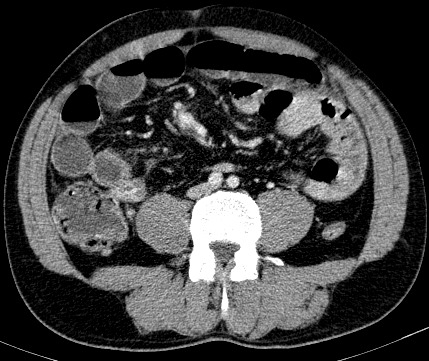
axial enhanced abdominal CT scan shows a cluster of jejunal loops in the Landzert’s fossa
